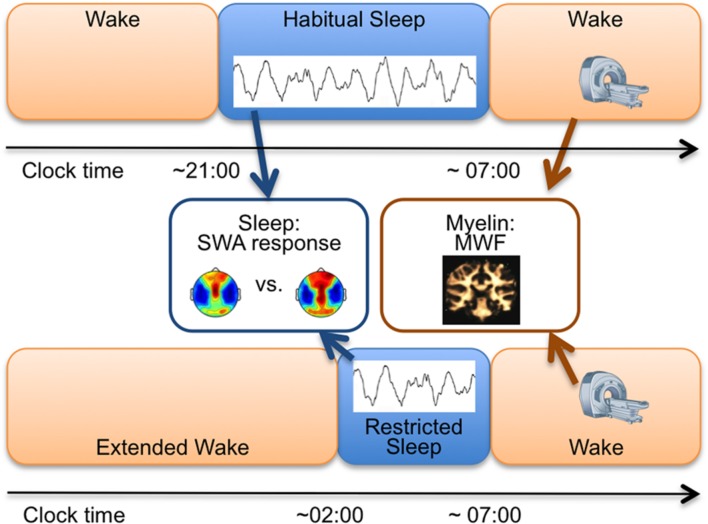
Study Protocol. Study measures for each subject involved: (i) One night of high-density EEG during habitual sleep (HS) and restricted sleep (RS; blue boxes) to assess slow-wave activity (SWA, 1–4.5 Hz) topography and to compare SWA between conditions and (ii) mcDESPOT magnetic resonance imaging scan in the morning either in the HS or the RS condition to assess myelin water fraction (MWF) masks. The two conditions were counterbalanced and separated by at least 7 days.
